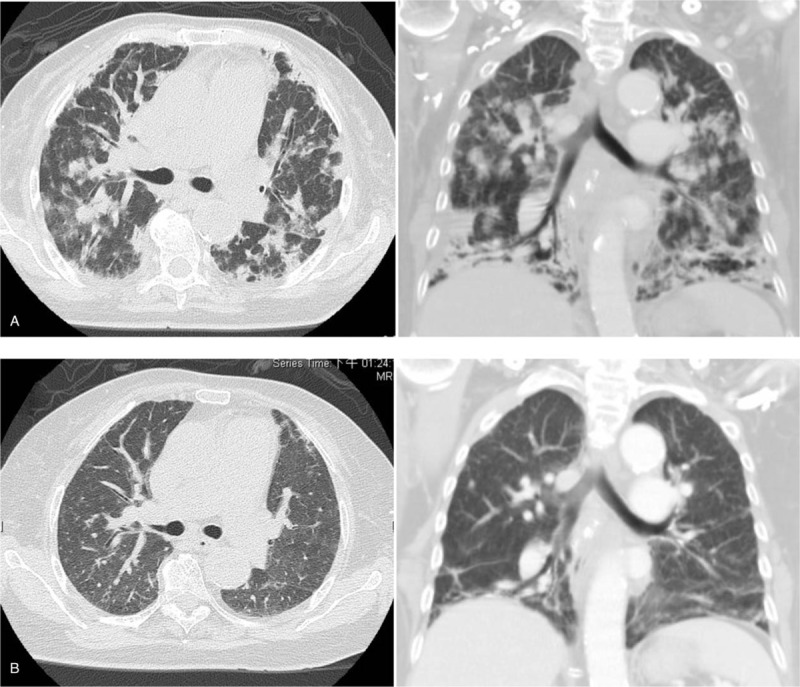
A computed tomography scan revealed: (A) diffuse peribronchial and subpleural consolidations in bilateral lungs with disease progression; (B) resolution of most infiltrates 3 months later.
